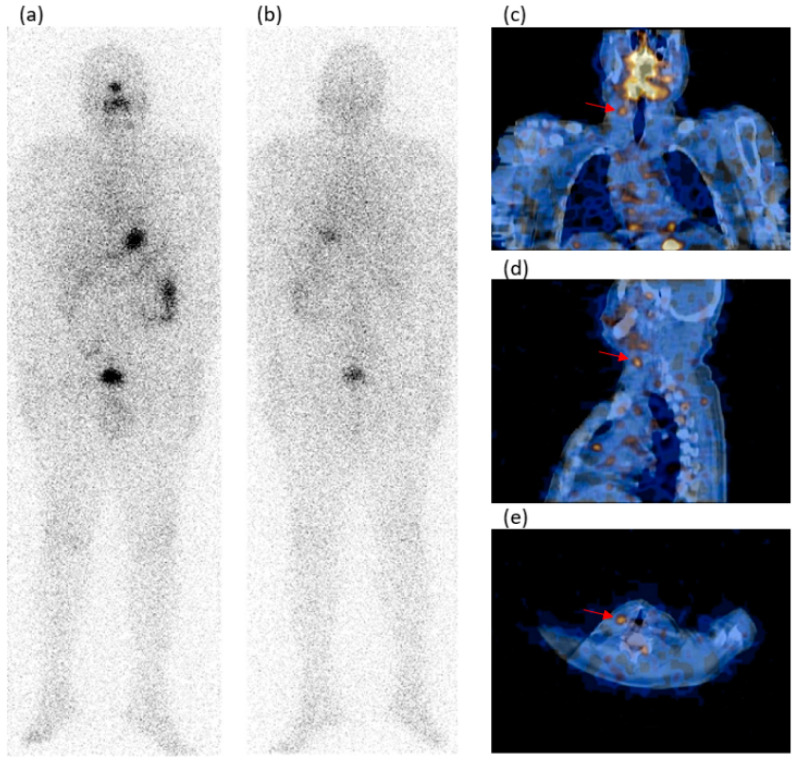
A 54-year-old male patient who underwent a total thyroidectomy and radioiodine ablation for a 30 mm T2 papillary carcinoma (PC) classic variant. During surgery, mETE and neck LN laterocervical metastasis were ascertained. During the follow-up, the WBS in anterior/posterior (a,b) views did not evidence pathologic radioiodine uptake foci. The SPECT/CT on the coronal/sagittal/transaxial (c–e) slides showed a radioiodine avid focus (red arrows) in the neck (right laterocervical region). This focus was classified as a metastatic lymph node and was submitted to a second surgery and radioiodine ablation. Thyroglobulin levels were in the hypothyroidism range before the present scintigraphy: 22 ng/mL; AbTg: absent.
